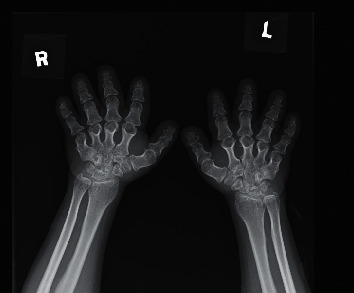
Patient's mother's X-ray hand demonstrating brachydactyly.
